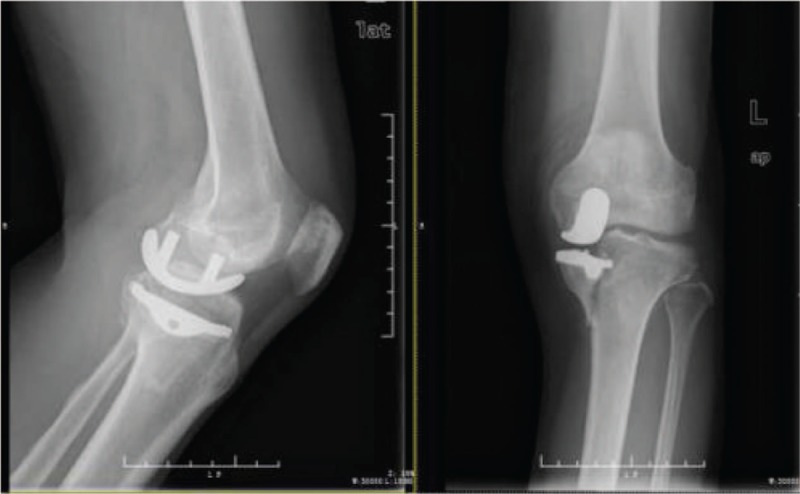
Preoperative radiograph showing medial malleolus fracture of the left knee, followed by a slight downward shift of the medial tibial plateau.
